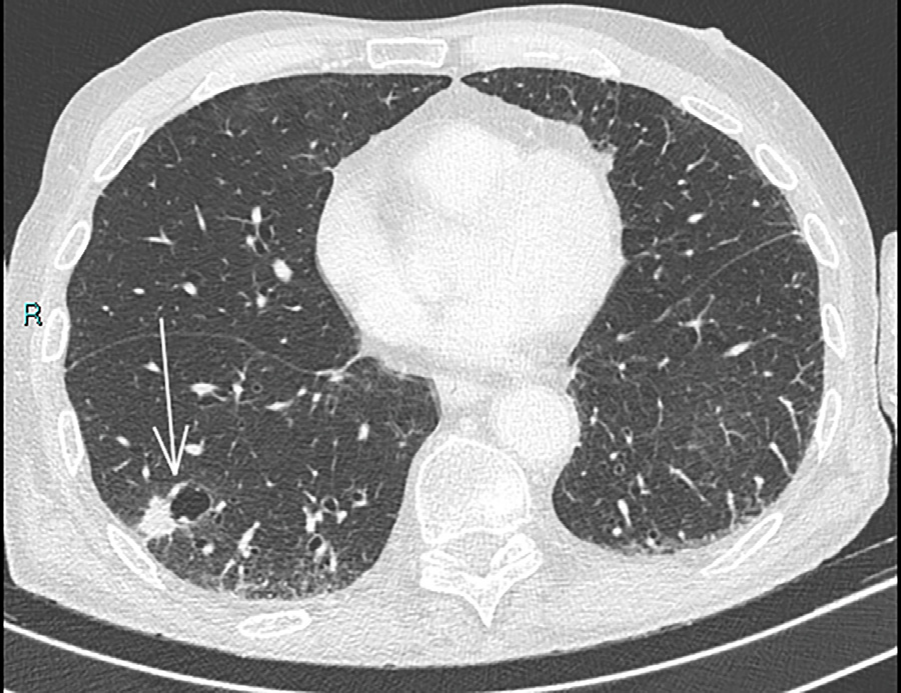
CT showing solid tissue with spiculated edges adjacent to a cystic formation (white arrow).
